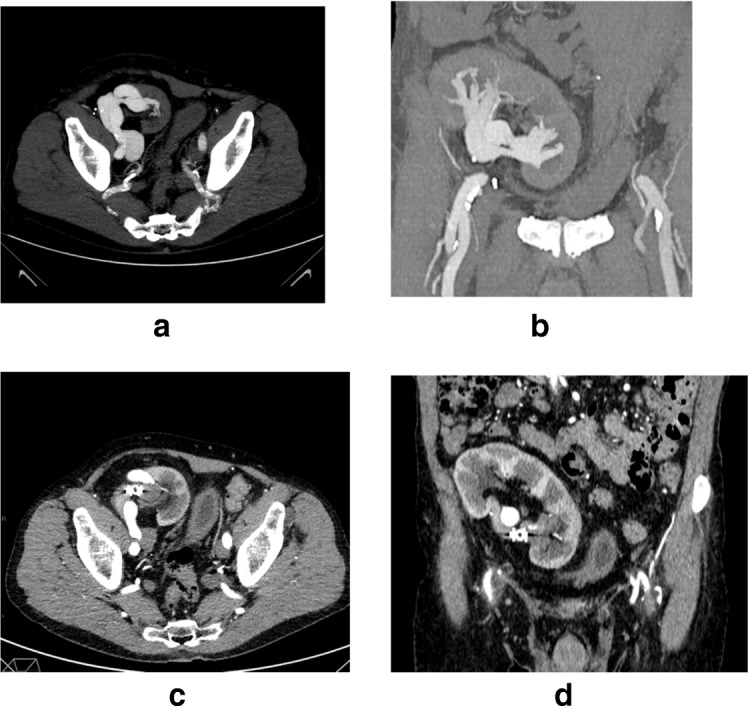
2-month follow up CT angiogram. Pre procedure axial (a) and coronal (b) and post procedure axial (c) and coronal (d) images prove successful closure of AVF with Amplazter plug and no perfusion defect
